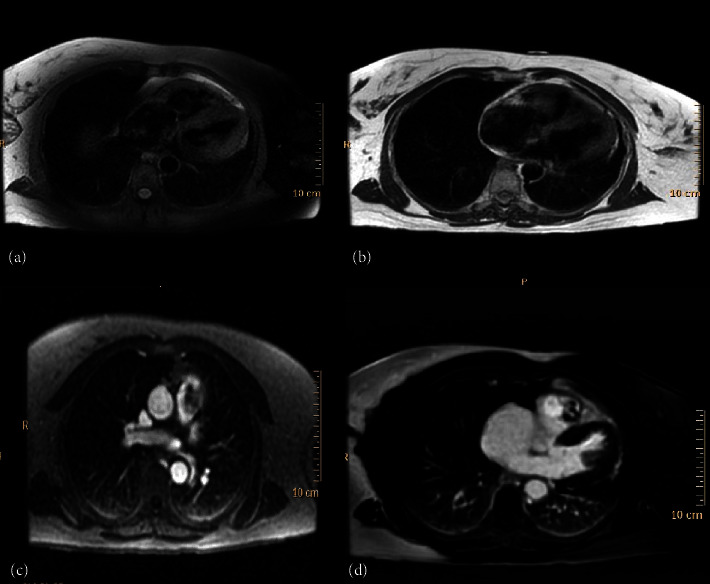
Cardiac magnetic resonance imaging shows RV mass. (a) shows RV mass, which is hypointense on T1 sequence, isointense on T2 (b), (c) shows poor first pass perfusion, and (d) shows heterogeneous uptake on late gadolinium enhancement. Abbreviations: RV: right ventricle.
